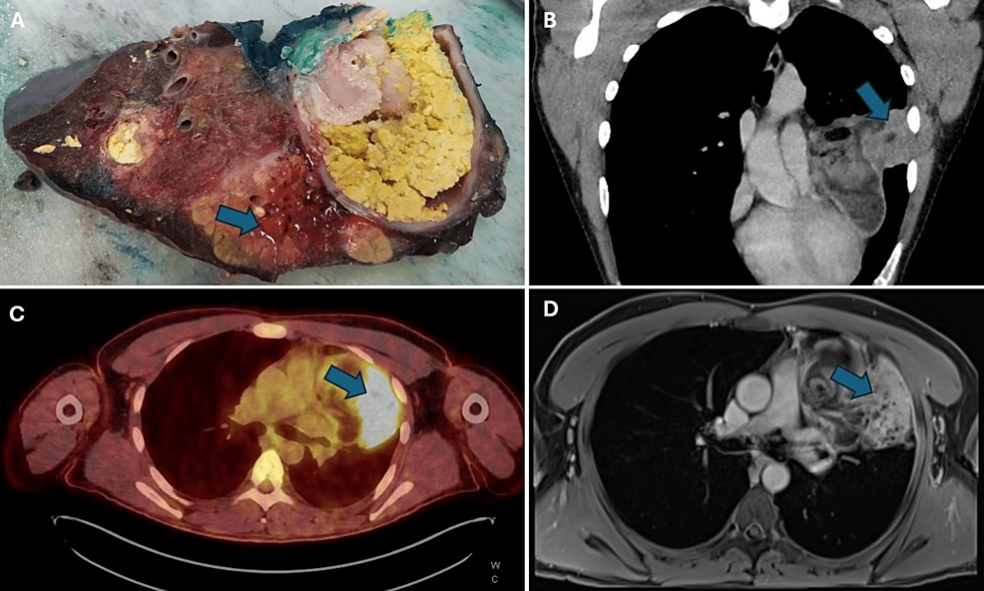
Macroscopic appearance and radiographic features of the lipoid pneumonia. There is a macroscopic display of signs of pneumonia (arrow) in the lung tissue with some fatty elements in the adjacent area (A). A retrospective analysis of the paracoronal multiplanar reconstruction (B) displayed communication between the teratoma and the lung (arrow). PET CT scan showed high metabolic activity in the lateral part of the mass which was indicative of lipoid pneumonia retrospectively (C). MRI T1 post-contrast pictures (D) showing pneumonia with some fatty elements in the bronchial tree (arrow).
